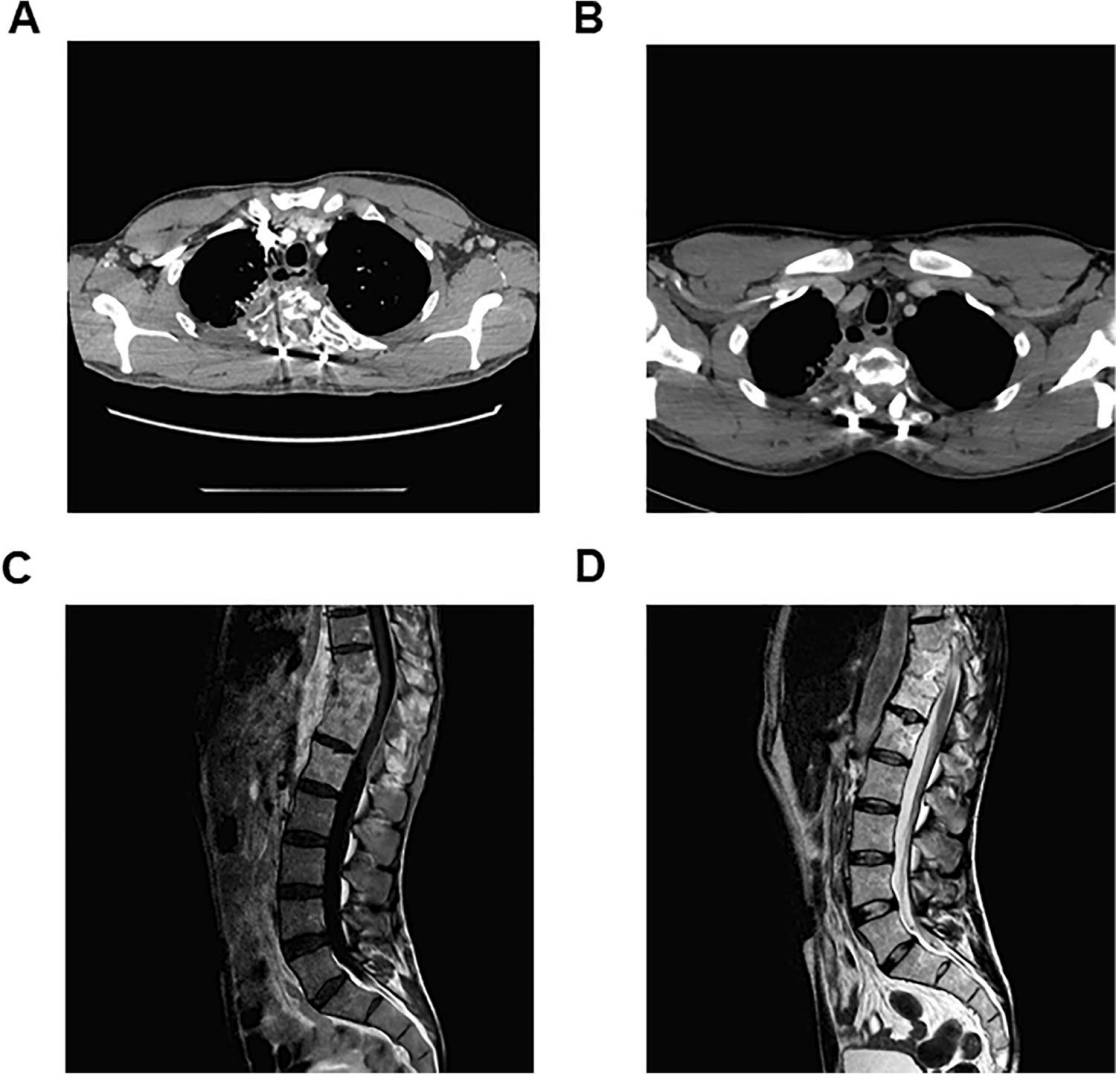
A PT1 CT chest: vascularized pathological tissue infiltrating lung, pleura, chest wall (rib and dorsal vertebrae), esophageal fistula. B PT1 CT chest: clear reduction of pathological tissue devoid of vascularization with spinal stabilization. Partial resolution of esophageal fistula. C PT2 MRI dorso-lumbar spine: spondylodiscitis (vertebral edema and multiple paravertebral fluid collections). D PT2 MRI dorso-lumbar spine: complete resolution of vertebral edema and fluid paravertebral collections
